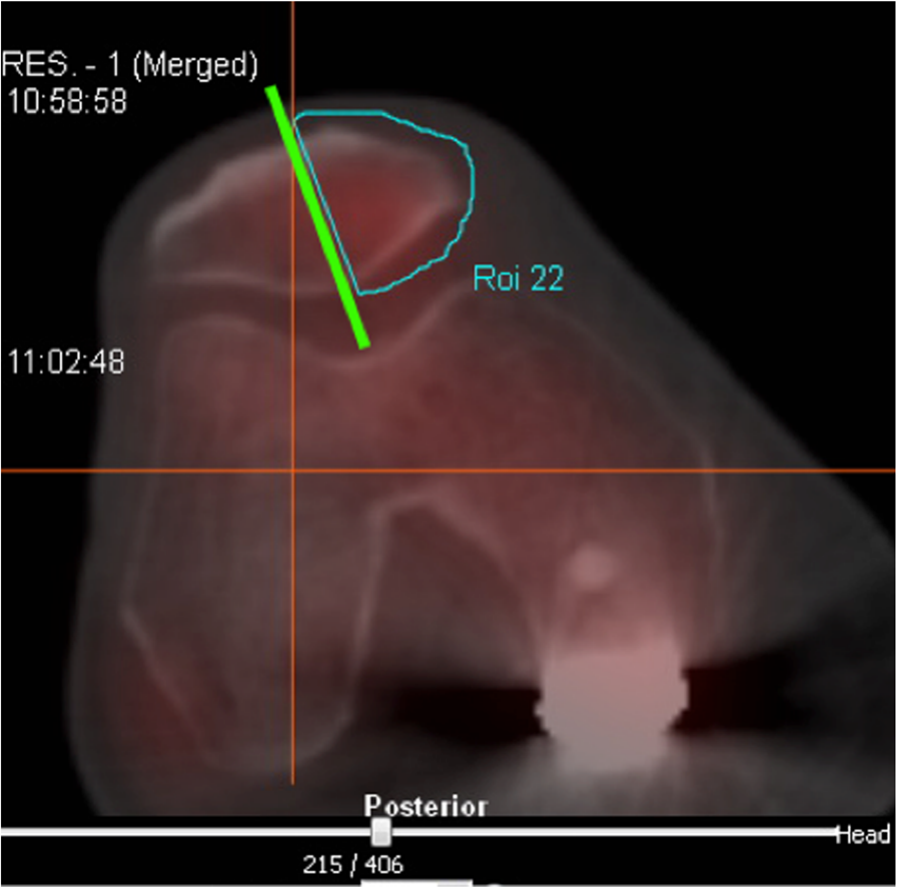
In transverse planes of the SPECT-CT fusion images regions of interests (ROIs) were defined manually. Here this process is shown for the medial facet of the patella. Many ROIs then comprised a volume of interest (VOI). Within the VOI the maximum tracer uptake was determined
